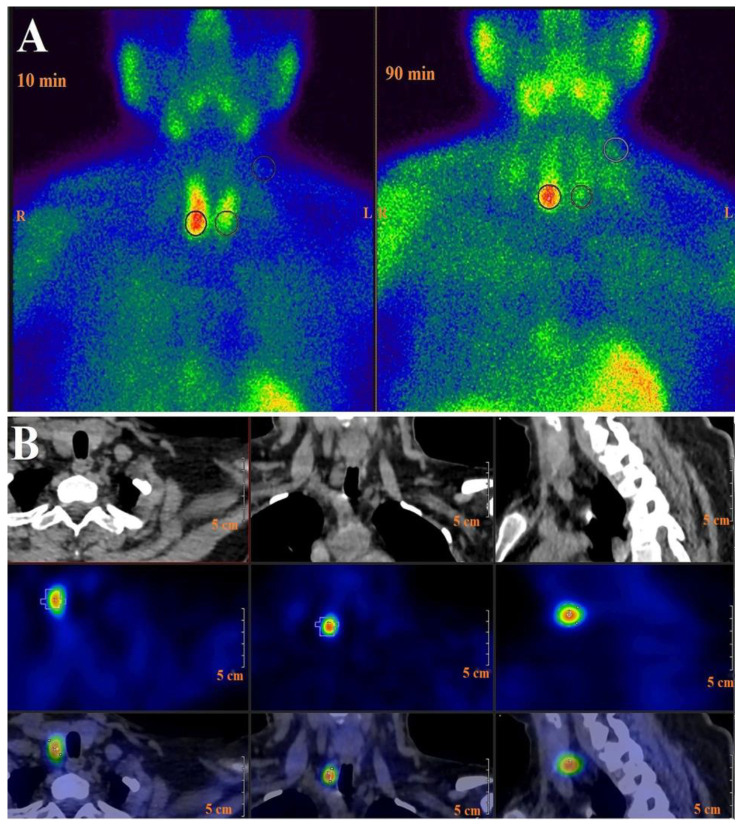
(A) Calculation of early lesion to background ratio (eLBR), early lesion to thyroid ratio (eLTR); delayed lesion to background ratio (dLBR) and delayed lesion to thyroid ratio (dLTR) from the region of interest (ROI). (B) Determination of parathyroid adenoma volume (Svol) from the SPECT imaging using semi-automated volume of interest option.
